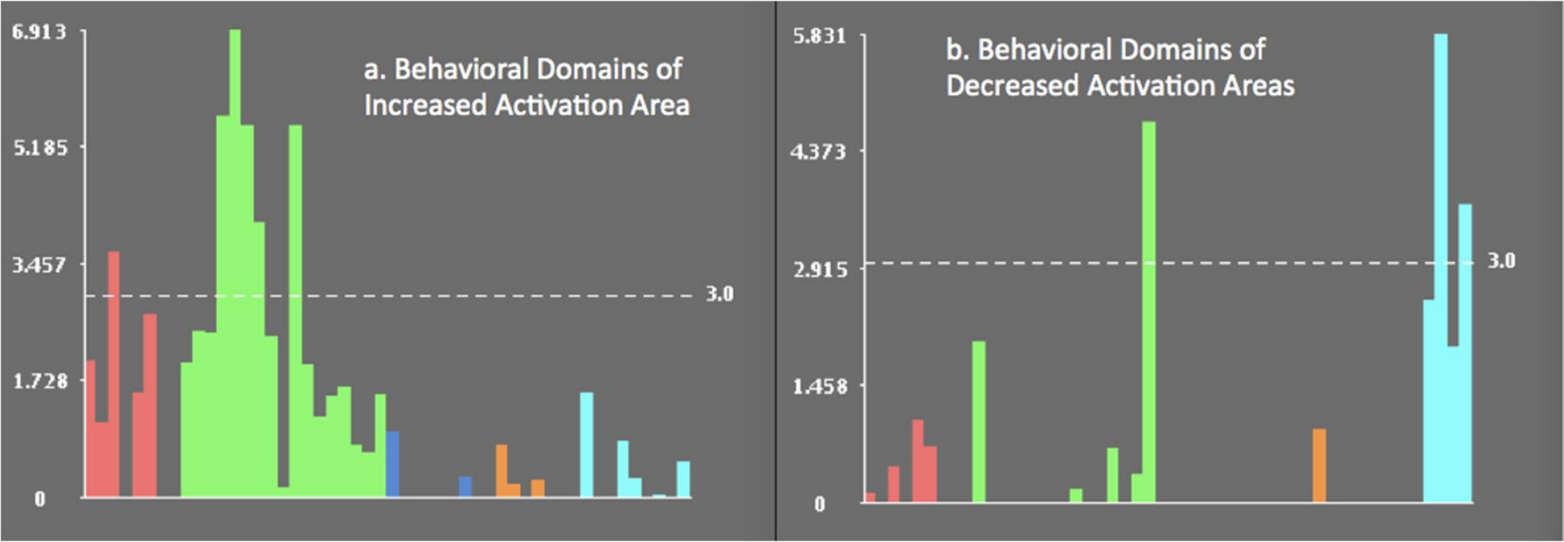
Histogram of the results of the behavioral analysis for regions implicated in CT. a. shows the behavioral domain Z-scores of regions of increased activation and b. shows the behavioral domain Z-scores of regions of decreased activation. Significant outcomes are on the dashed line. Blue: perception, Green: cognition, Red: action
